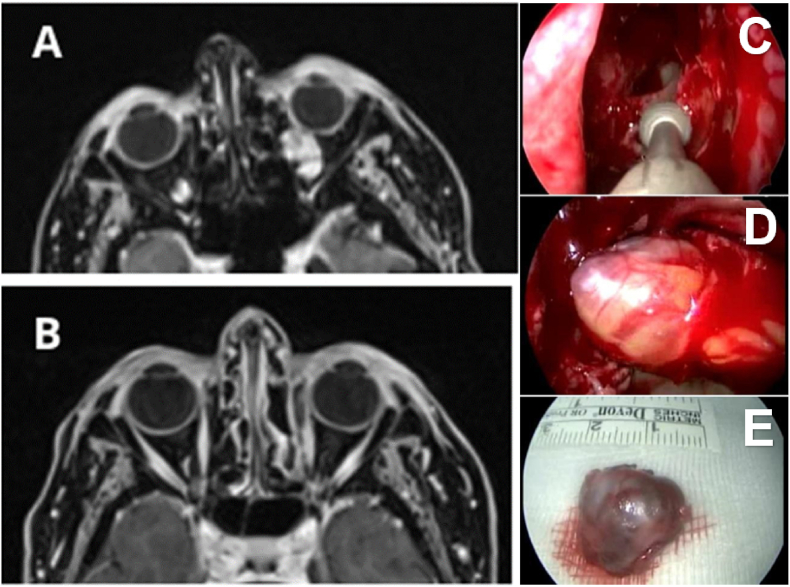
(A) Preoperative MRI showing the presence of a mass in the inferomedial quadrant of the orbit with rich enhancement (B) Postoperative MRI showing total excision of the lesion (C, D) endoscopic endonasal surgery with ethmoidectomy. (E) Operative piece following excision. Source: Archive image IRCCS San Matteo di Pavia ENT department.
